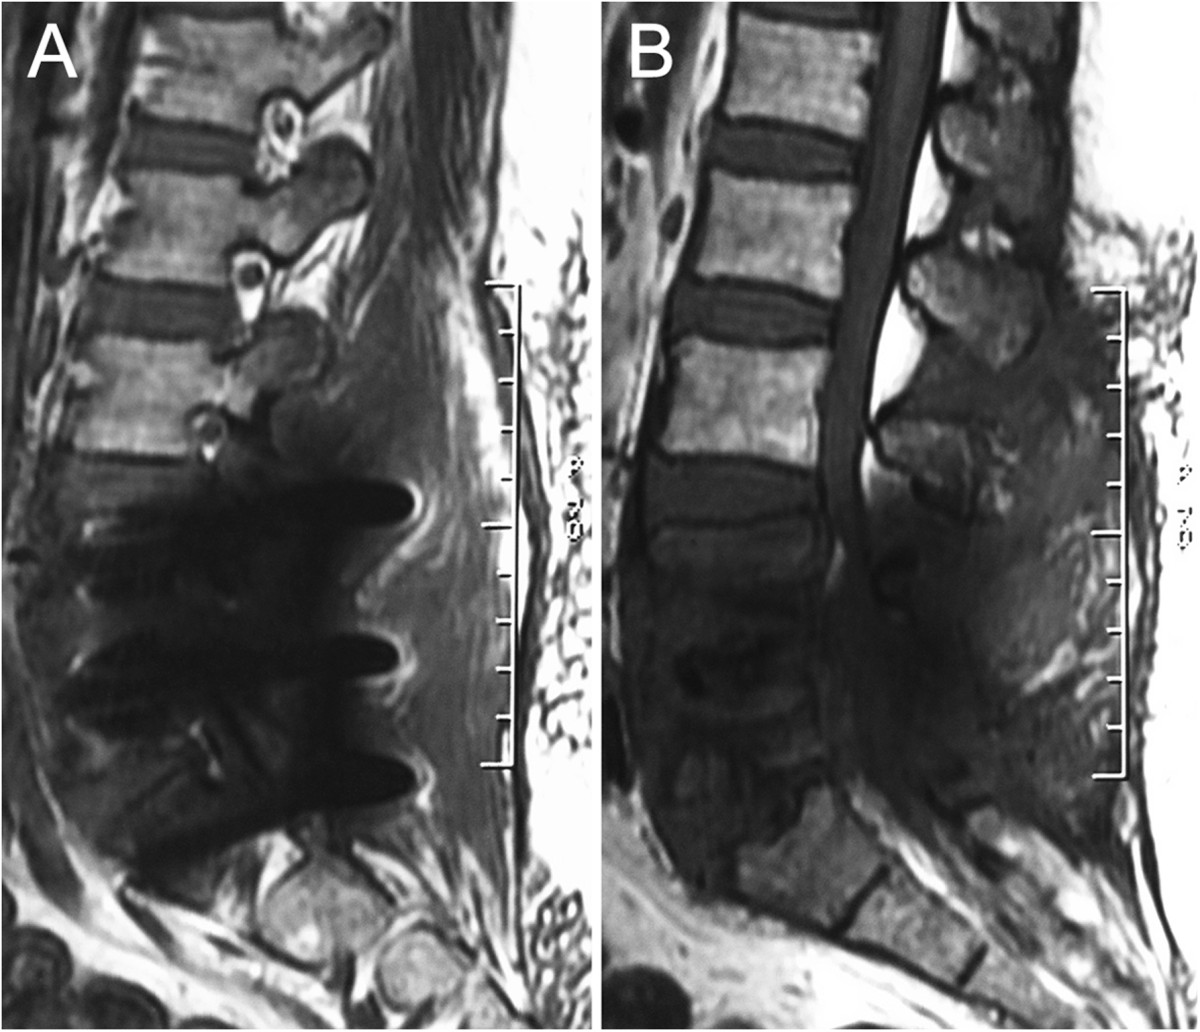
Sagital, 3.0 T, T1-weighted magnetic resonance image(MRI) demonstrate the swelling adjacent soft tissue (A) and “cloud sign” in the adipose layer (B).
